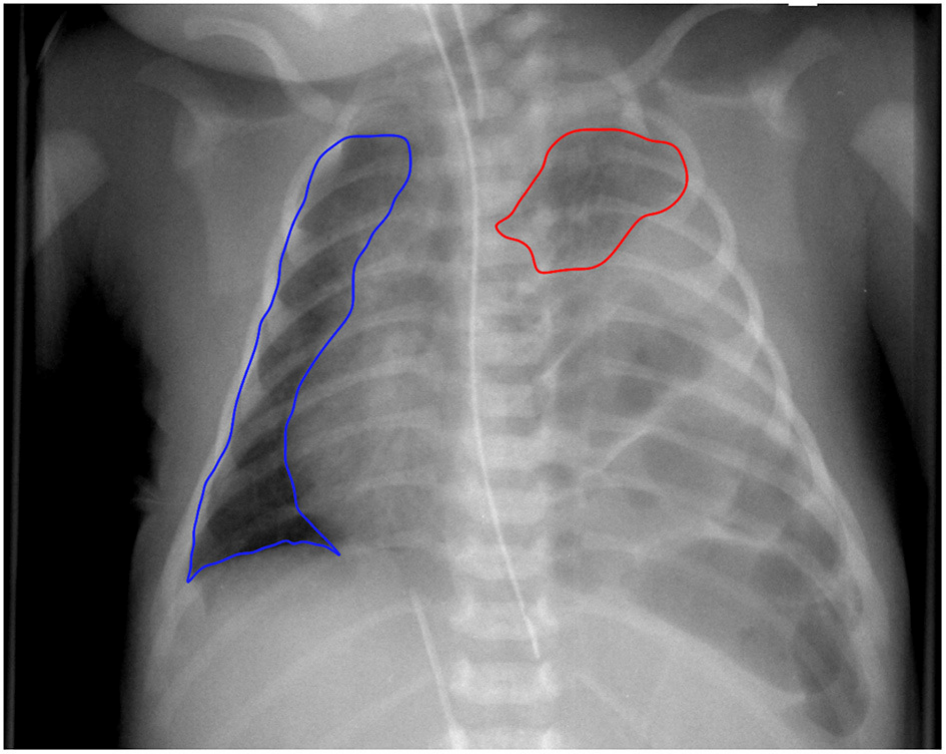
Delineation of chest radiographic thoracic area. Example of a newborn with left-sided congenital diaphragmatic hernia. CRTA was calculated as the sum of area of ipsilateral and contralateral lung. Segmentation was performed manually.
